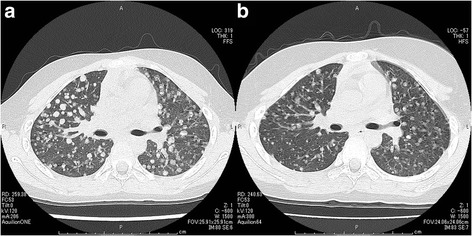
Radiographic changes in lung metastases. a Chest computed tomography scan showing innumerable metastases. b In 2 month after surgery and resume sorafenib showing regression of lung metastases
